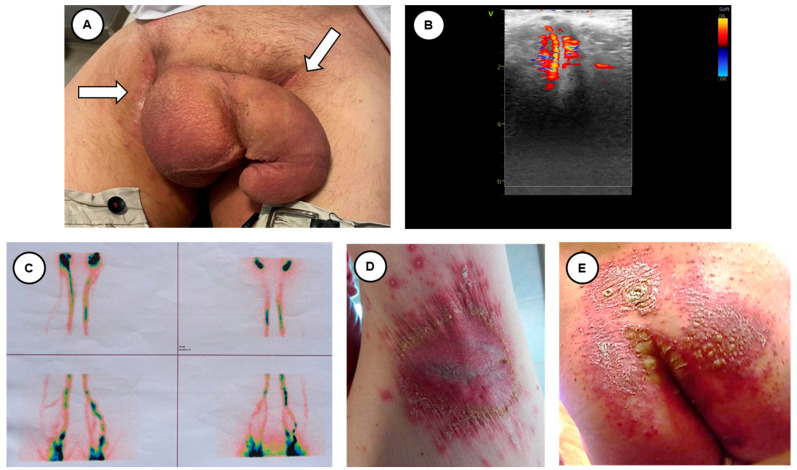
A healthy 54-year-old man previously presented to vascular surgeons with a 4-year history of swelling of the penis and scrotum was scheduled for ultrasound evaluation in the angiology office. At presentation, there was a giant enlargement of the penis and scrotum, without swelling of the legs (A). The penile ultrasound was negative for vascular abnormalities and/or thrombotic complications (B). A diagnosis of chronic lymphatic disease was suspected; thus, a lymphoscintigraphy was performed (C). This test was normal, showing a good visualization of major lymphatics. The patient had a history of psoriasis, with a documented previous event of flexural psoriasis, as shown in (D) (axillary fossa) and (E) (left and right gluteus and intergluteal cleft), also involving his genitals with secondary infection 4 years before. Since that infection, his genitals progressively increased in size, and despite medical treatment and different surgical evaluation, the patient’s symptoms did not resolve, with marked disability related to walking and sexual activity. The diagnostic work-up for filariasis was performed as soon as the genital enlargement started, with negative results (direct detection in the blood, antigen detection and molecular diagnosis by PCR). The patient reported that, before experiencing penis enlargement, an acute phase of psoriasis occurred with signs of infection, as diagnosed by the family practitioner, and because of that, empirical antibiotic treatment was started. Taking into account the negative results of the test for filariasis, and the absence of vascular obstruction (as shown by the Doppler evaluation) as well as lymphatic obstruction (as shown by the lymphoscintigraphy), the role of inverse psoriasis in generating this process was postulated.
